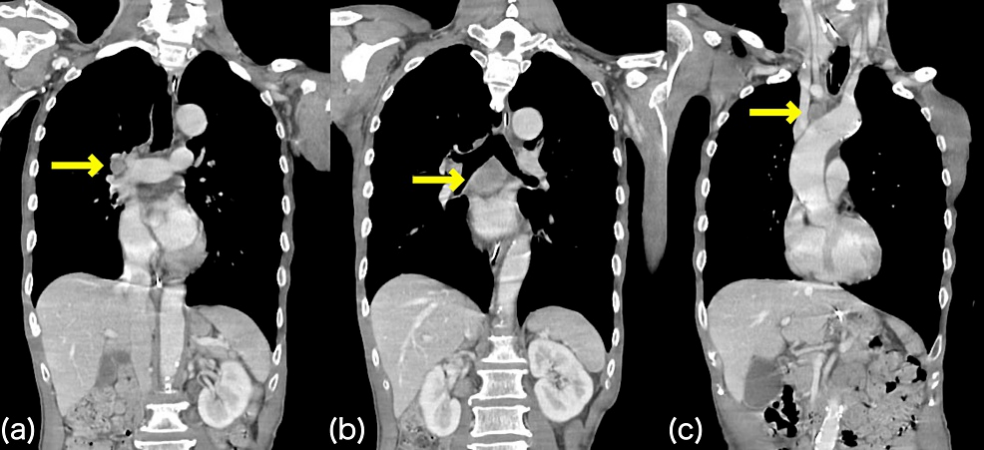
Recurrence eight months after onset Although chest computed tomography scans did not show any obvious mass, multiple lymphadenopathies were observed, and the diagnosis of recurrence was made. (a) Right hilar lymphadenopathy; (b) paratracheal lymphadenopathy; and (c) right upper paratracheal lymphadenopathy.
